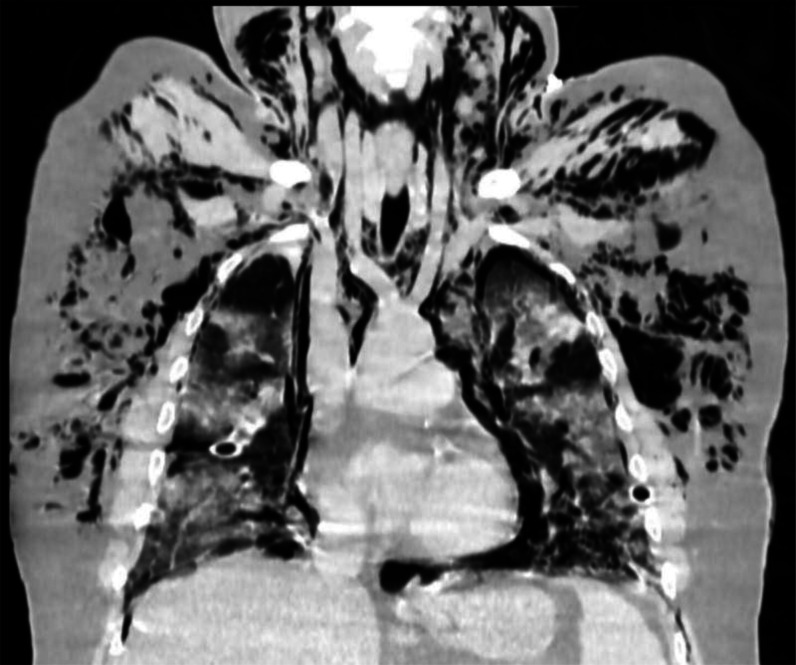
Coronal HRCT window image showing air foci extending above the root of the aorta into the neck region suggestive of pneumomediastinum.
